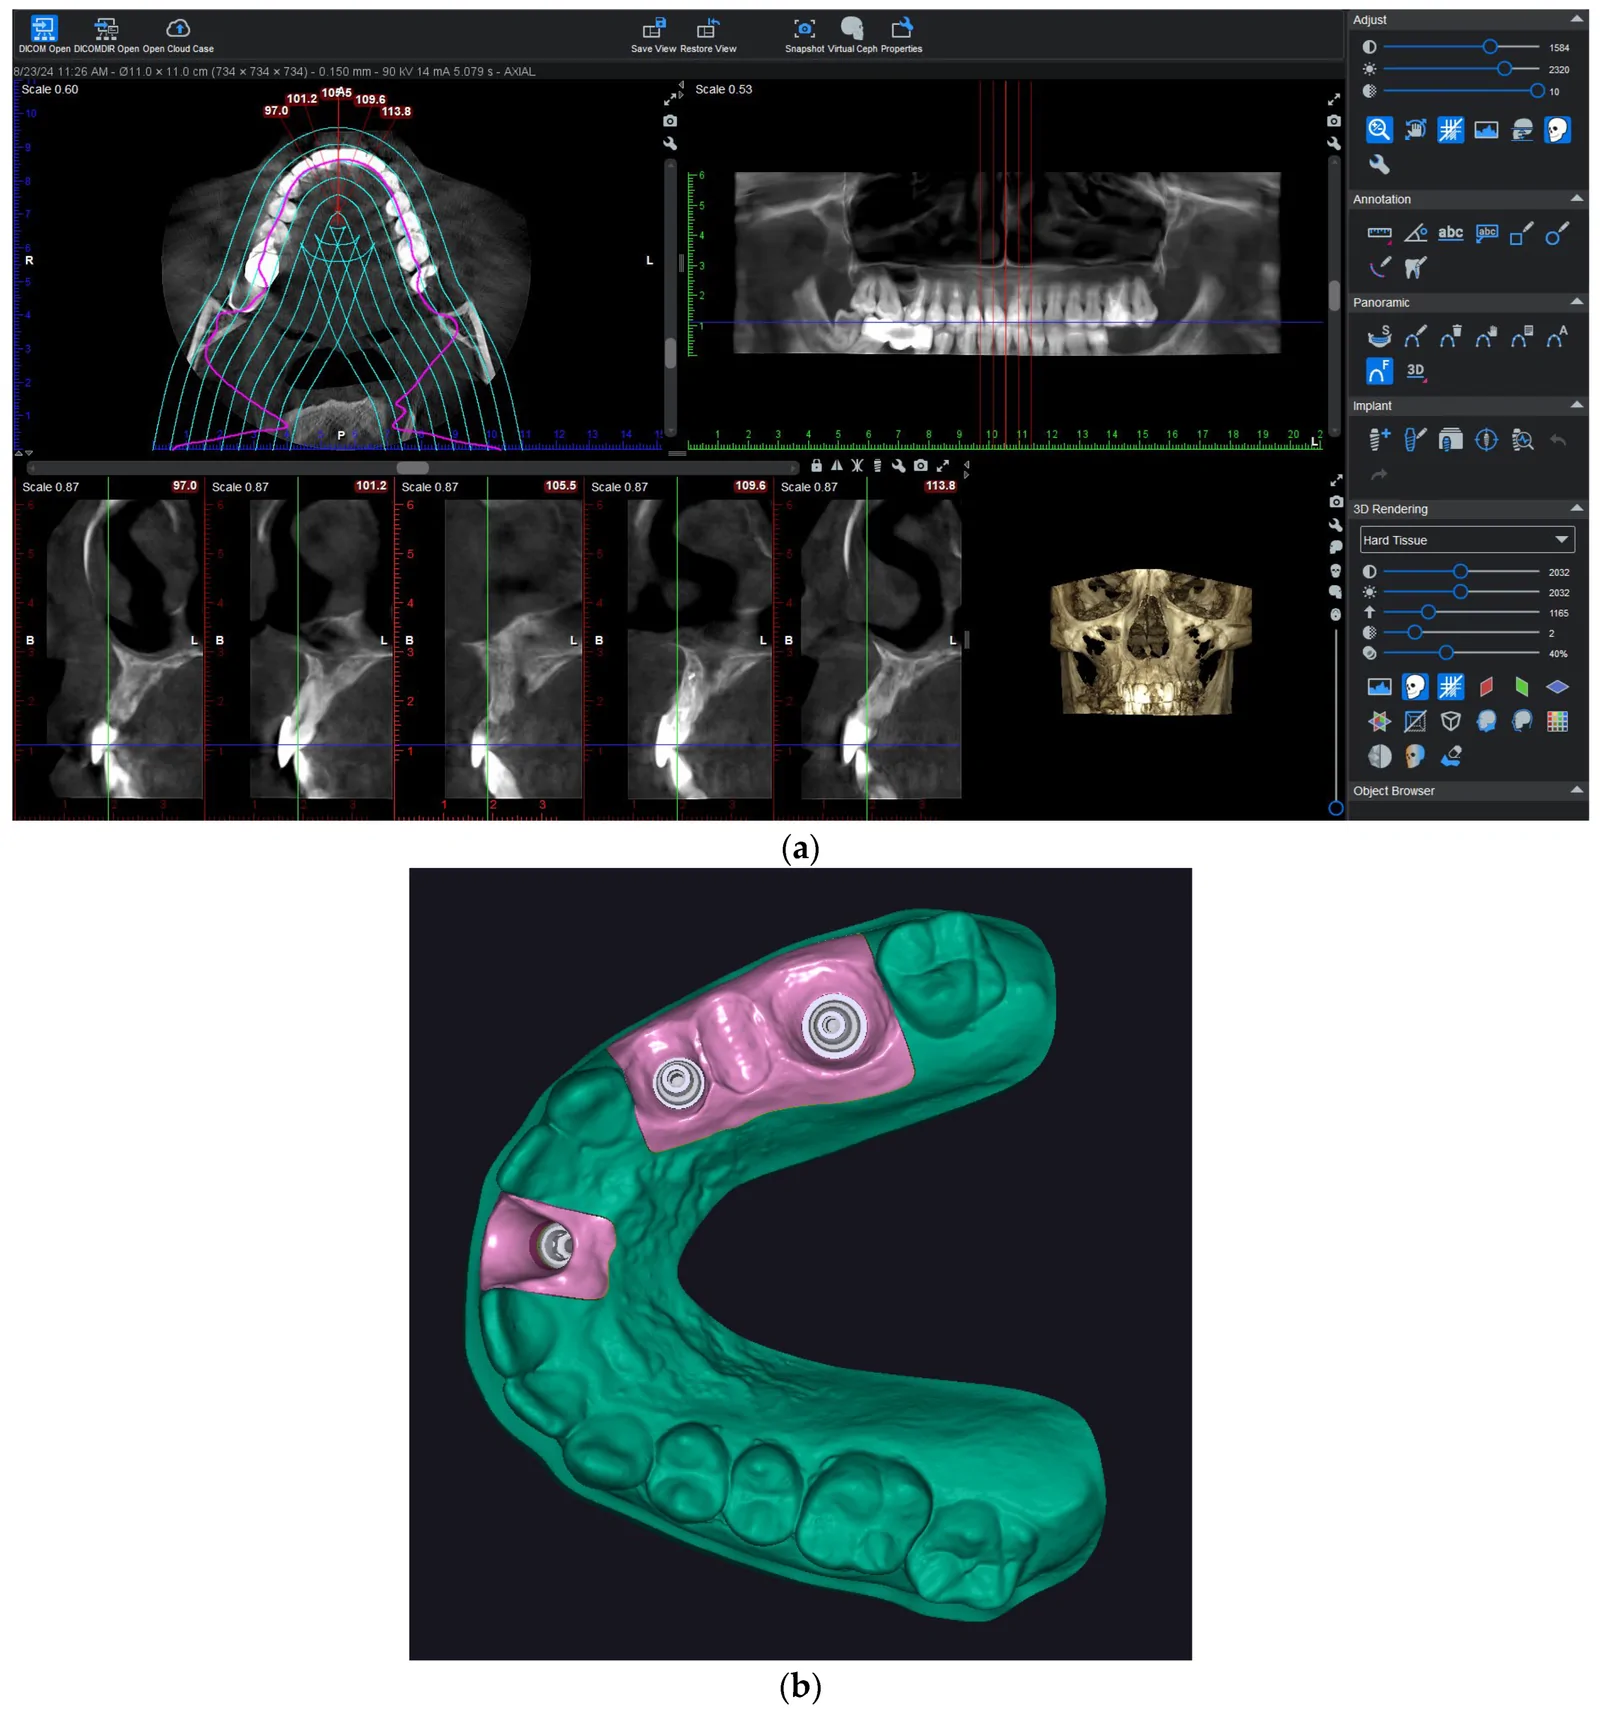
CBCT-based implant planning in Planmeca Romexis (a) and prosthetic surface design in Exocad (b).
